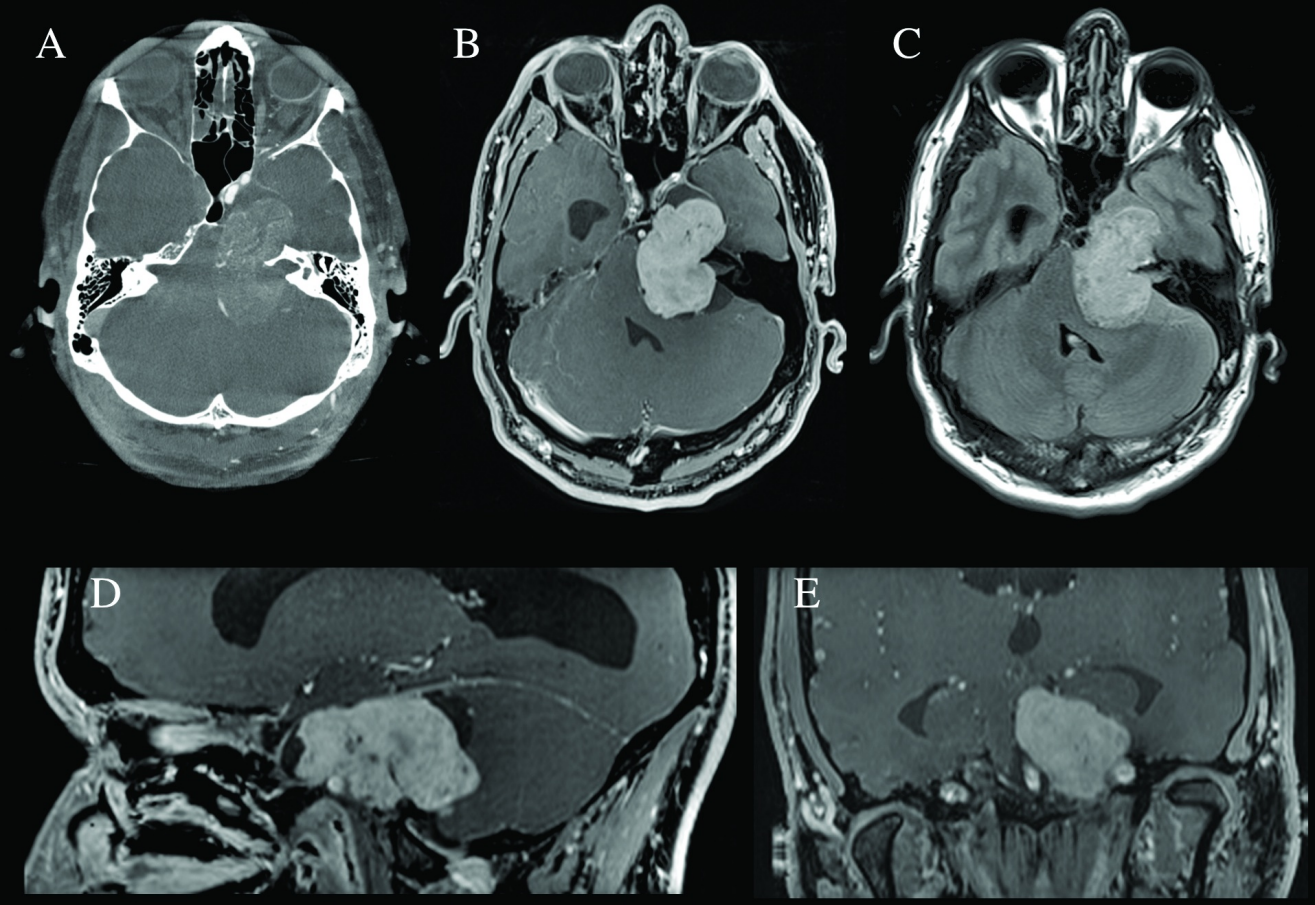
Preoperative imaging A. Axial computed tomography (CT) scan (bone windows) showing a lesion at the level of the left Meckel´s cave with significant petrous bone remodeling. B. Axial T1-weighted magnetic resonance imaging (MRI) with gadolinium showing a homogenously enhancing extra-axial lesion, with significant mass effect on the brain stem, fourth ventricle, and left temporal lobe, with an enlarged right ventricular temporal horn. C. Axial fluid-attenuated inversion recovery (FLAIR) weighted MRI showing lack of edema in brainstem, cerebellum and left temporal lobe. D. Sagittal T1-weighted MRI with gadolinium showing the tumor´s middle and posterior fossa involvement as well as the anatomical relationship with the petrosal portion of the left carotid artery and the enlarged lateral ventricle. E. Coronal section demonstrating an enlarged supratentorial ventricular system and tumor adjacent to the bilateral carotid arteries.
